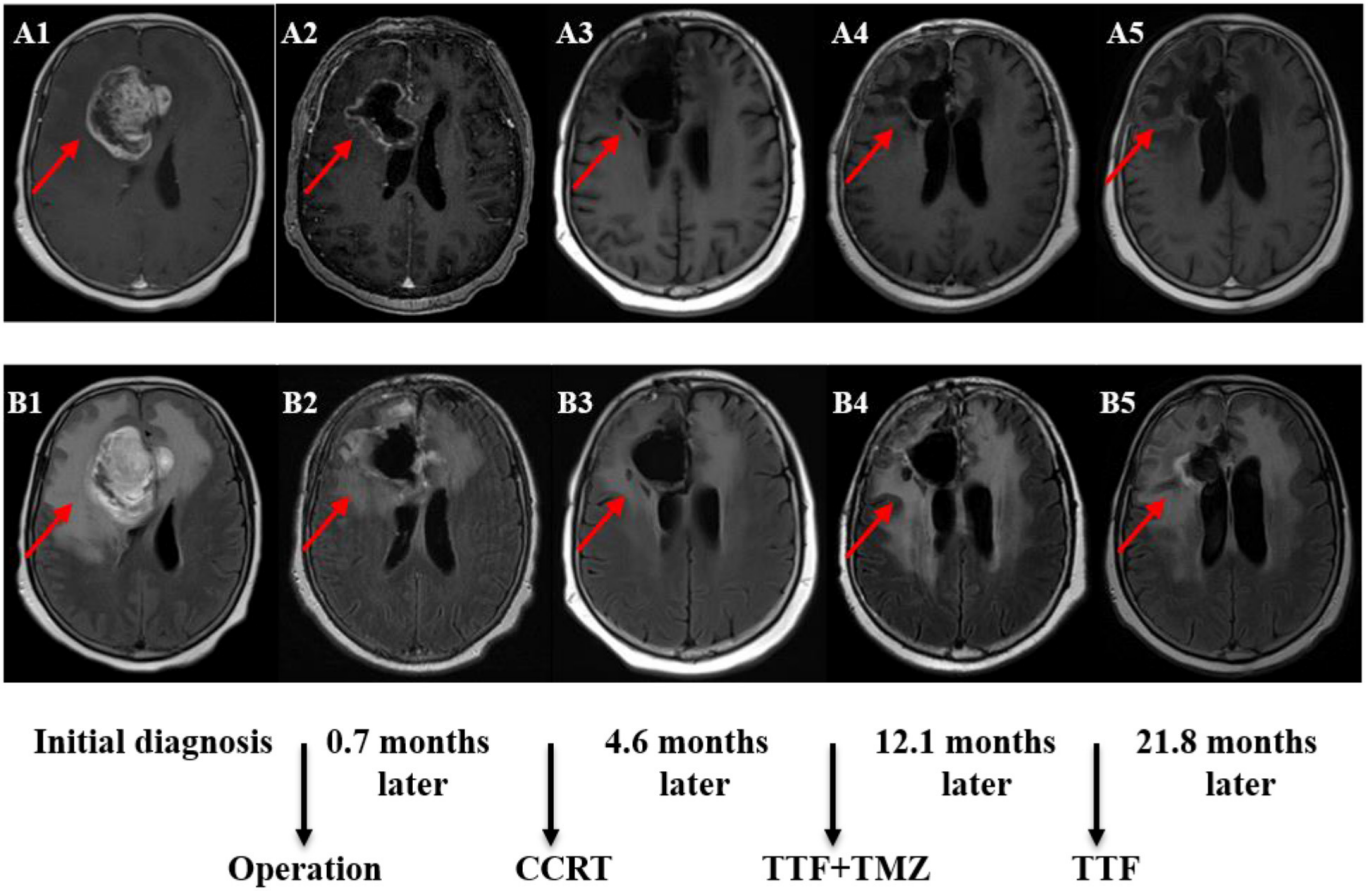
MRI (Magnetic Resonance Imaging) changes of newly diagnosed glioblastoma (GBM). (A1–A5) Contrast-enhanced MRI; (B1–B5) MRI-Flair. MRI images followed-up every 2 months before and after treatment. Only some of the images were exhibited. The arrow indicates the tumor and tumor bed. This patient has followed-up for 35.4 months with a stable disease.
